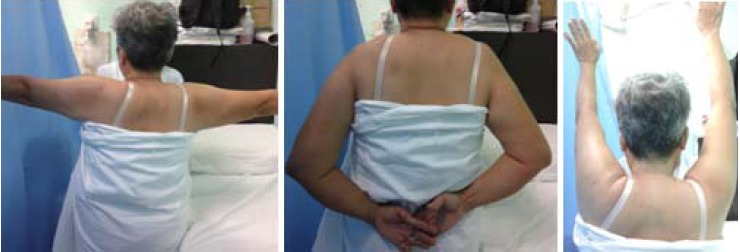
Fig. 4: Preoperative X-ray showing not much acromioclavicular joint narrowing(A) Postoperative, distal clavicle resection.(B) F4:
